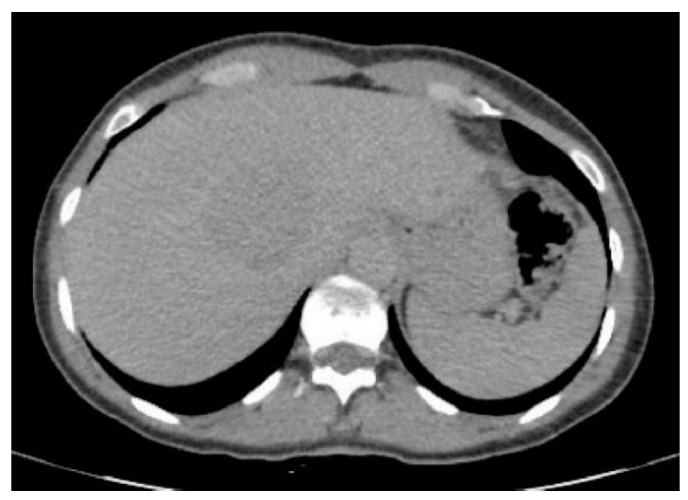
Computed tomography of the patient from Figure 1—“washing out” the contrast—the lesion appears in the delayed phase less attenuated compared to the liver parenchyma.
